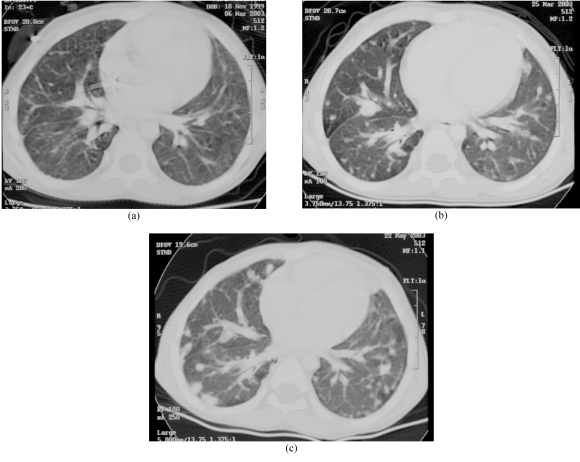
A four-year-old boy with sacrococcygeal endodermal sinus tumour. Blood culture grew *Peiciliomyces lilacinus*. (a) CT lungs showed tiny nodular lesions, presumed involvement of lungs by fungus. (b) After two weeks of anti-fungal therapy, CT showed the lung lesions had increased in size and number. (c) CT lungs after two months of continuous anti-fungal therapy showed more and larger lesions, which were likely to be metastases.
